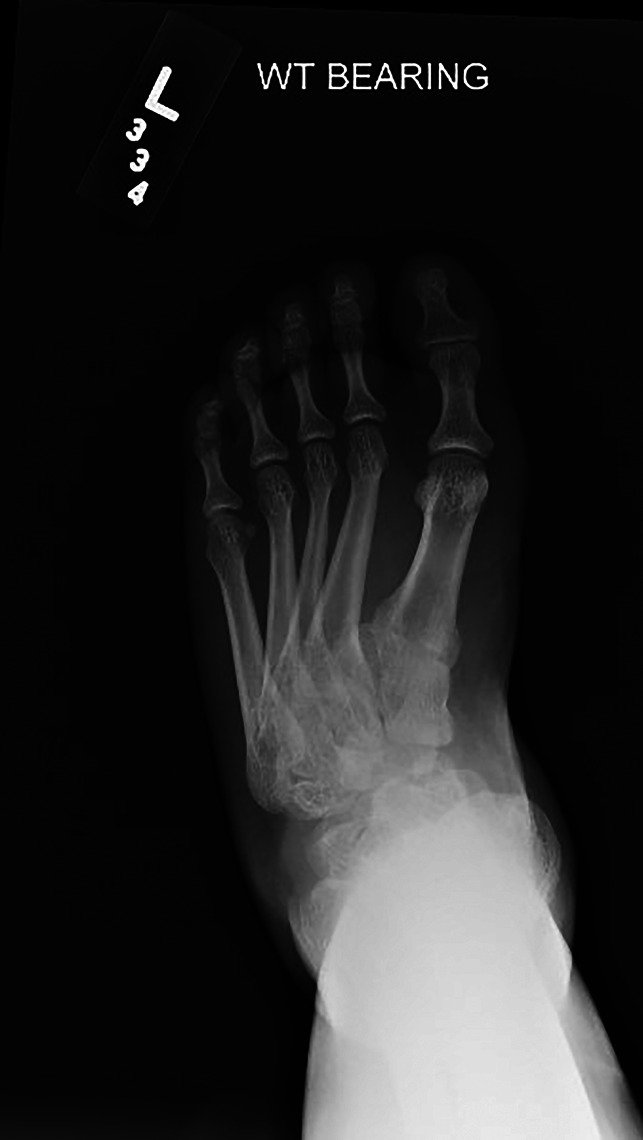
Anteroposterior preoperative radiograph of the left foot.
